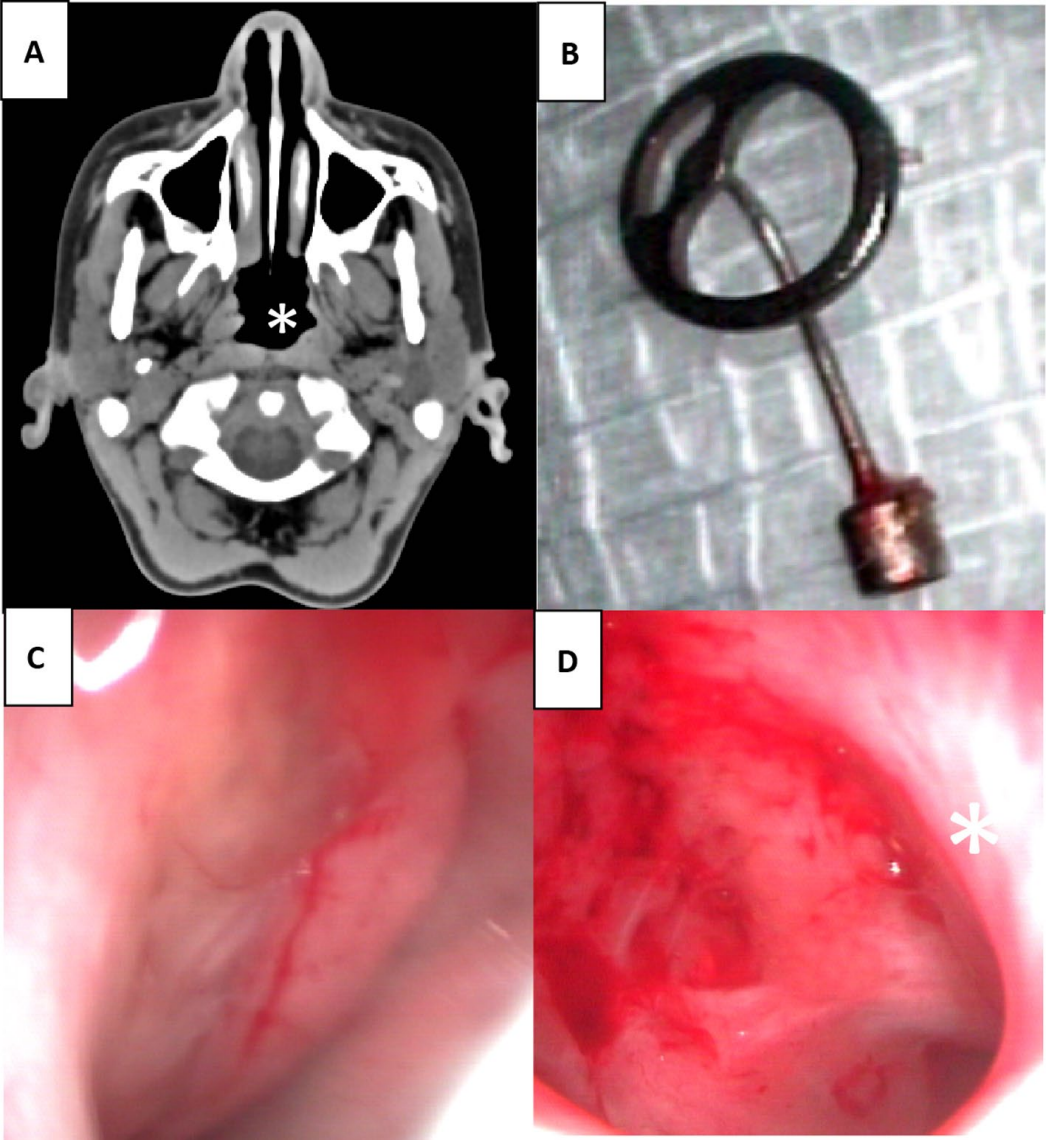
A Axial view of temporal CT showed complete deformity of the left Eustachian tube orifice (*). A 14-year-old male Caucasian patient underwent adenoidectomy at the age of 1 year and revision adenoidectomy at the age of 2. At the age of 3 years, he developed a left-sided cholesteatoma requiring 9 prior revision surgeries. B The left middle ear total ossicular chain prosthesis was severely bent due to the long-term negative pressure caused by POET. C and D The right orifice was not as severely damaged as the left orifice (*). This case required revision surgery for after reocclusion
